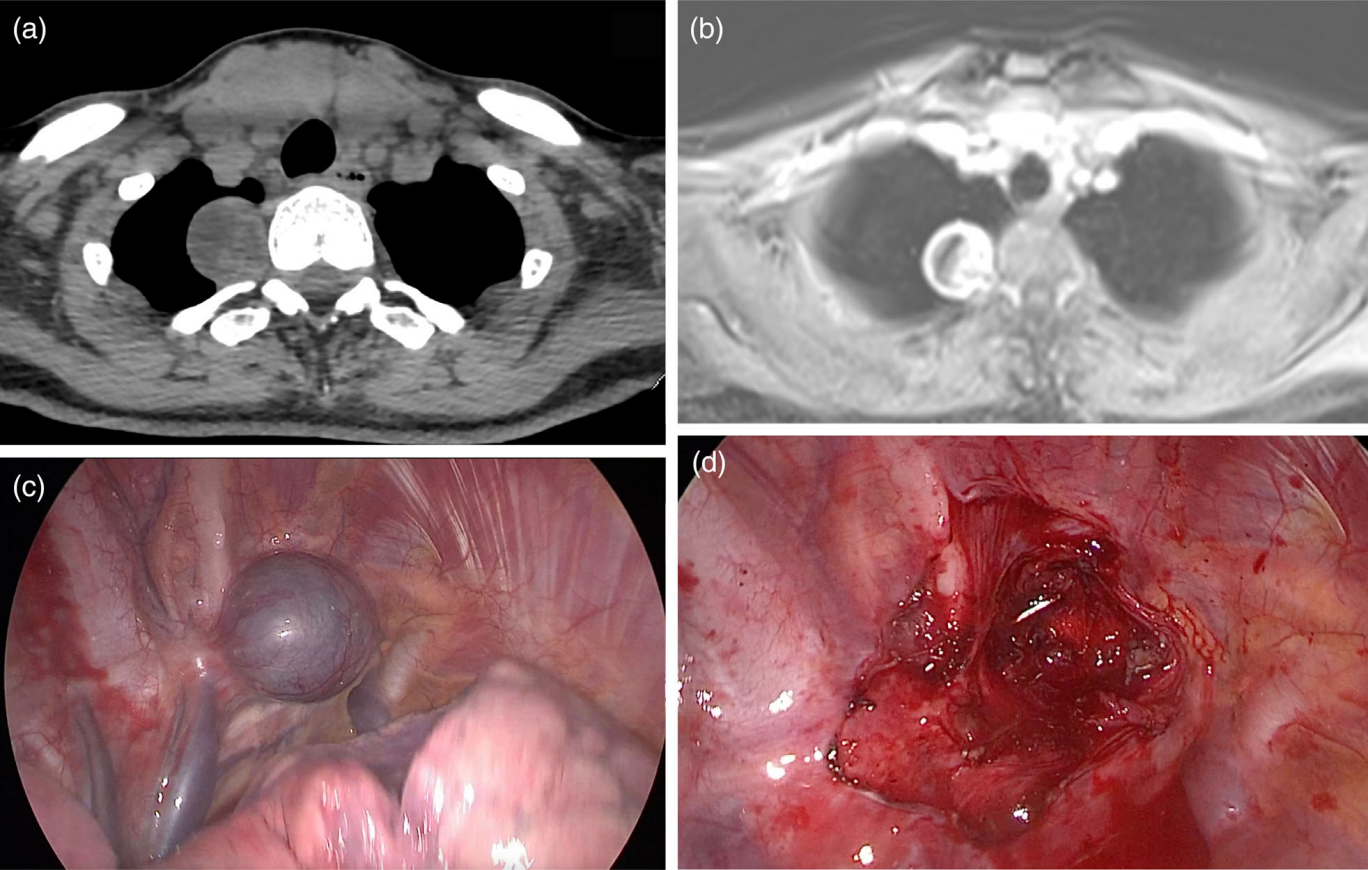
A 26‐year‐old woman with a symptomatic intrathoracic neurogenic tumor (ITN) on the right T2/3. Computed tomography (CT) scan and magnetic resonance imaging (MRI) did not show a spinal extension of the tumor (a, b) that was removed by right video‐assisted thoracoscopic surgery (VATS) (c, d).
